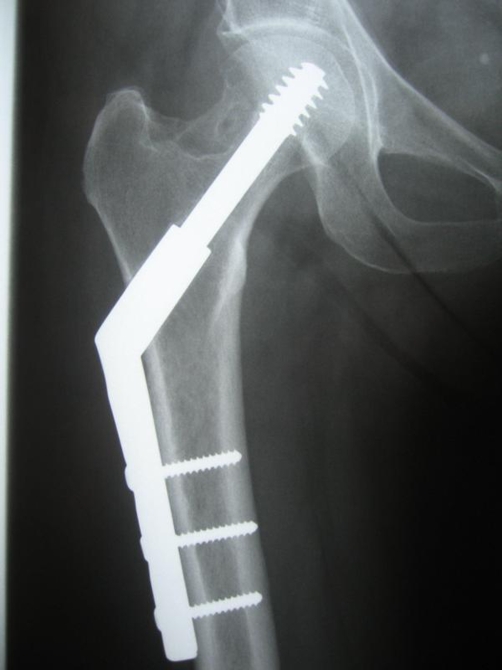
Plain radiography one-year post-operatively.
